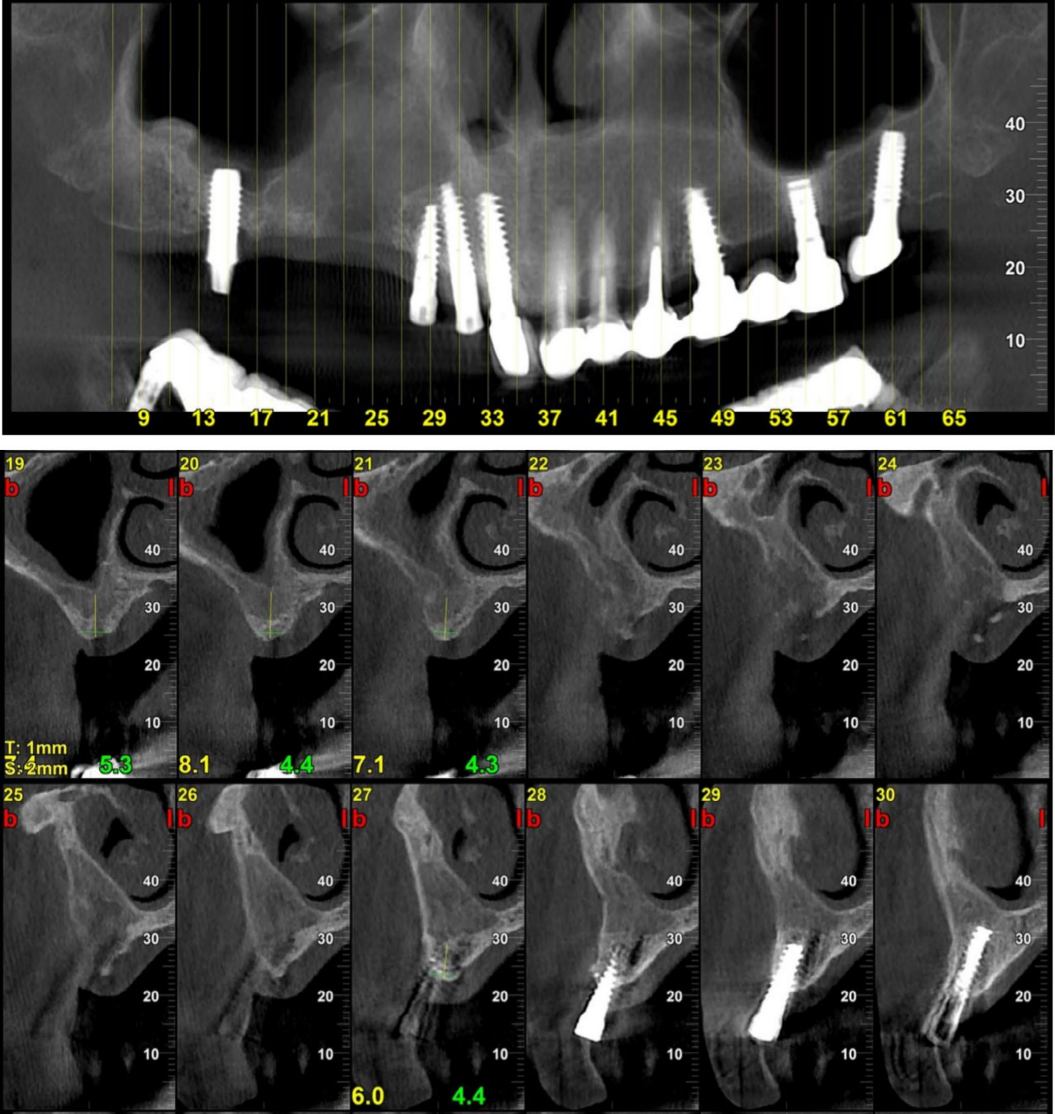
CBCT imaging at 9 months follow‐up post marginal maxillectomy and GBR—Case 3. Panoramic reconstruction from CBCT and axial views reveals a new bone formation on the right posterior maxilla filling the residual space.
